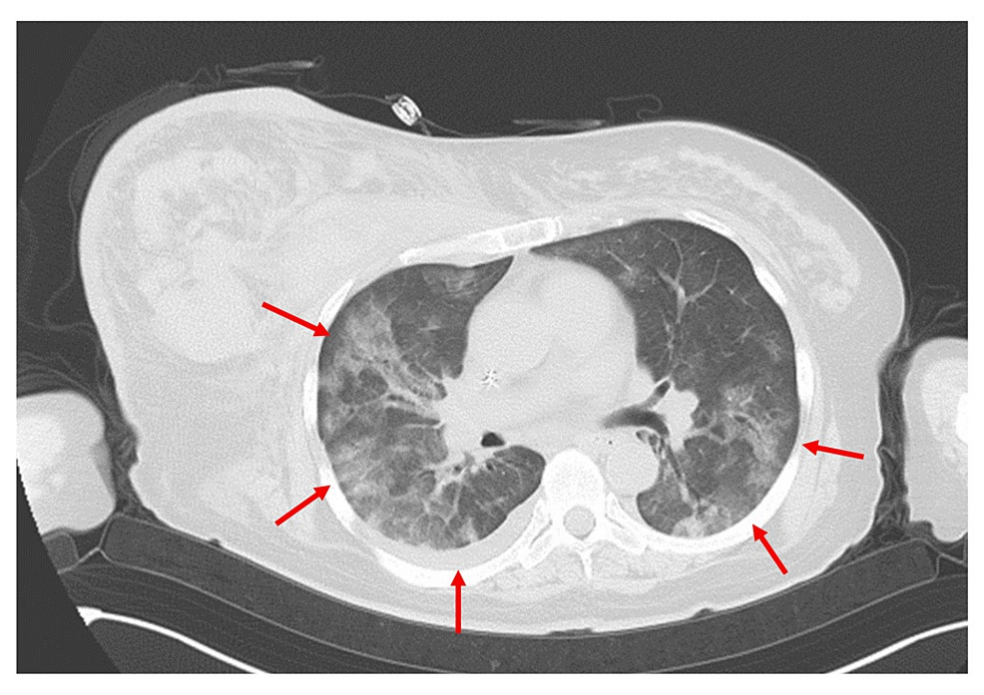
CT image of the patient on day 247. Bilateral diffuse ground-glass opacities were observed in the pulmonary fields (arrows).
